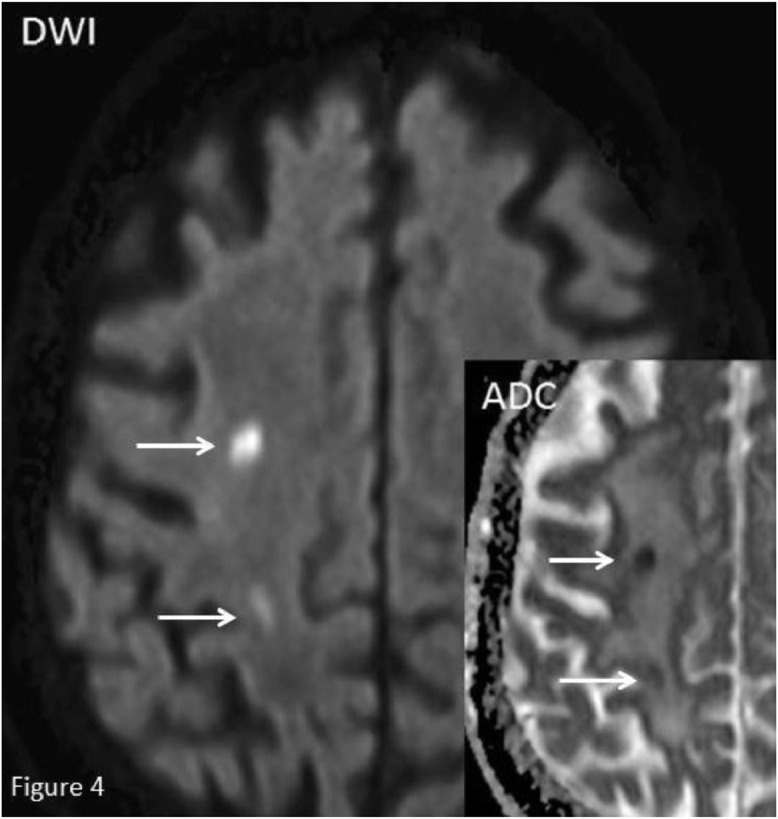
MRI Diffusion Weighted Imaging (DWI) and Applied Diffusion Coefficient (ADC) in axial plan view showing 2 small acute infarctions in the Anterior Cerebral Artery-Middle Cerebral Artery (ACA-MCA) watershed zone of the right cerebral hemisphere. The infarcts are shown using white arrows.
